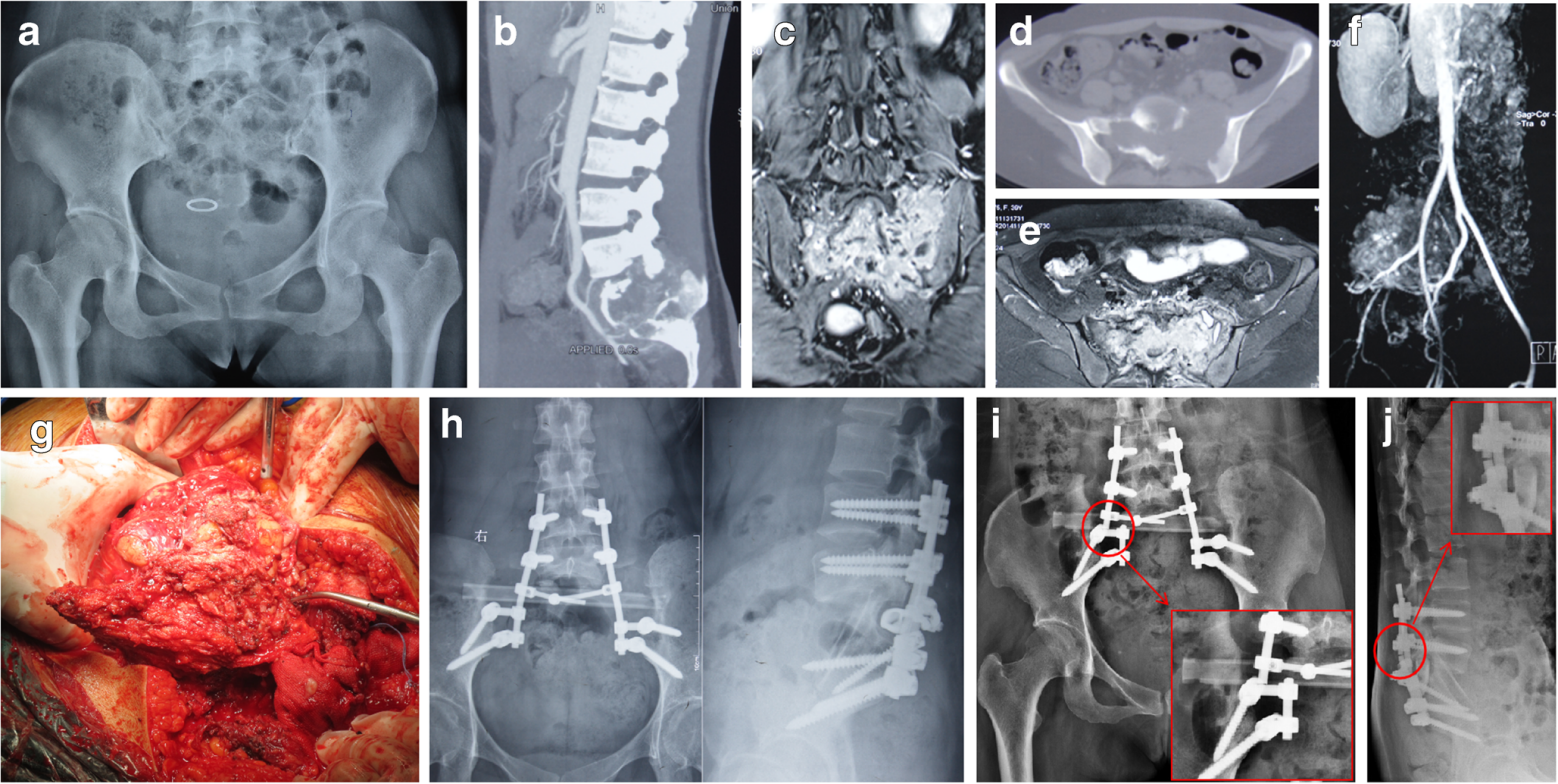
A 39-year-old female patient with an invasive giant cell tumour of the bone. a Pre-operative radiograph showing osteolytic destruction of the sacrum. b, d Pre-operative CT showing osteolytic bone destruction in the S1–S3 regions, with multilocular pores and bone crests inside. c, e T2-WI MRI shows mixed high signals and low signals in the bone crests. f Angiography showing abundant blood supply of the tumour. g Total sacrectomy. h One-year post-operative radiograph showing good fusion of the allograft, stable internal fixation, and no loosening or fracture of screws and connecting rods. i, j Two-year postoperative radiograph showing fracture of the right connecting rod. CT, computed tomography; WI, weighted image; MRI, magnetic resonance imaging
